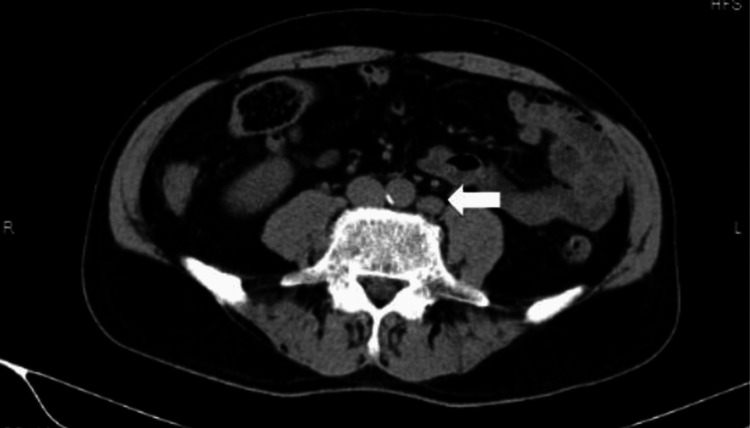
CT scan imaging of the abdomen at initial diagnosis Arrow head: swelling paraaortic lymph node
